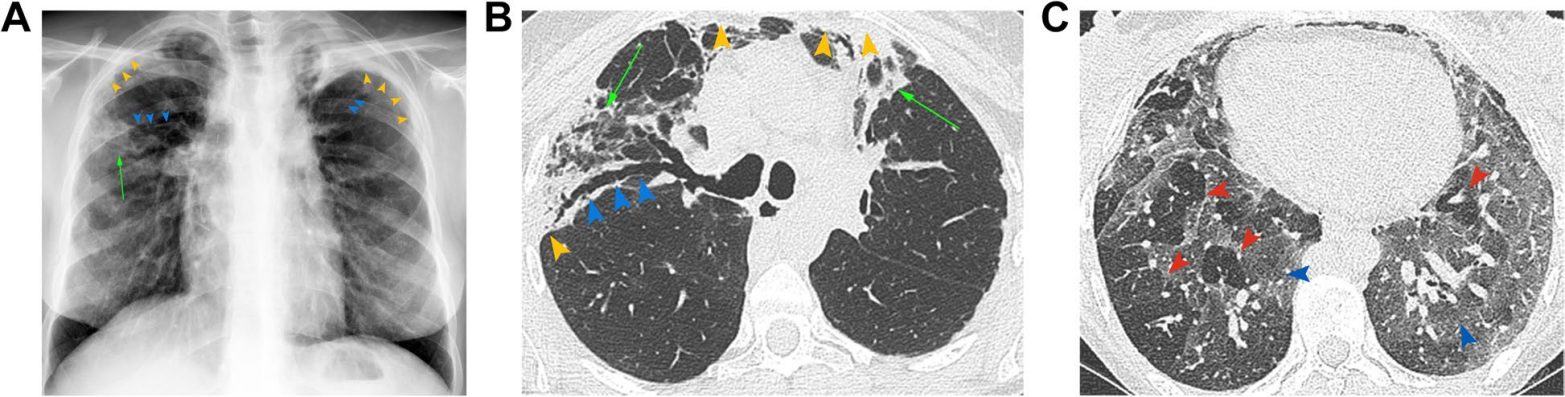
CLAD with mixed BOS/RAS features in a 37-year-old female 13 years out from lung transplants for cystic fibrosis. A Frontal chest radiograph 9 months after Covid-19 infection in this patient with persistent dyspnea and ventilatory defect on PFTs shows apical predominant pleural thickening (yellow arrowheads), bronchiectasis (blue arrowheads) and confluent fibrosis (green arrow). B Axial CT image (lung window) at the upper lobes (B) again shows areas of pleural thickening (yellow arrowheads) with subpleural confluent fibrosis (green arrow) and traction bronchiectasis (blue arrowheads). Findings are typical of pleuroparenchymal fibroelastosis. C Axial static end-expiratory CT image at the level of the lower lobes (lung window) shows lobular air trapping (red arrowheads) with intervening dense normal lung (blue arrowheads), representative of small airways disease and indicative of BOS
